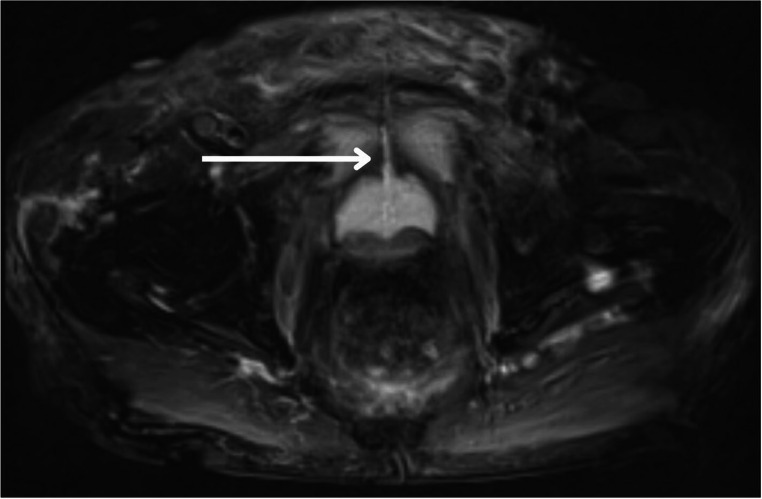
Pelvic MRI demonstrating fistulous tract between urethra and pubic symphysis
